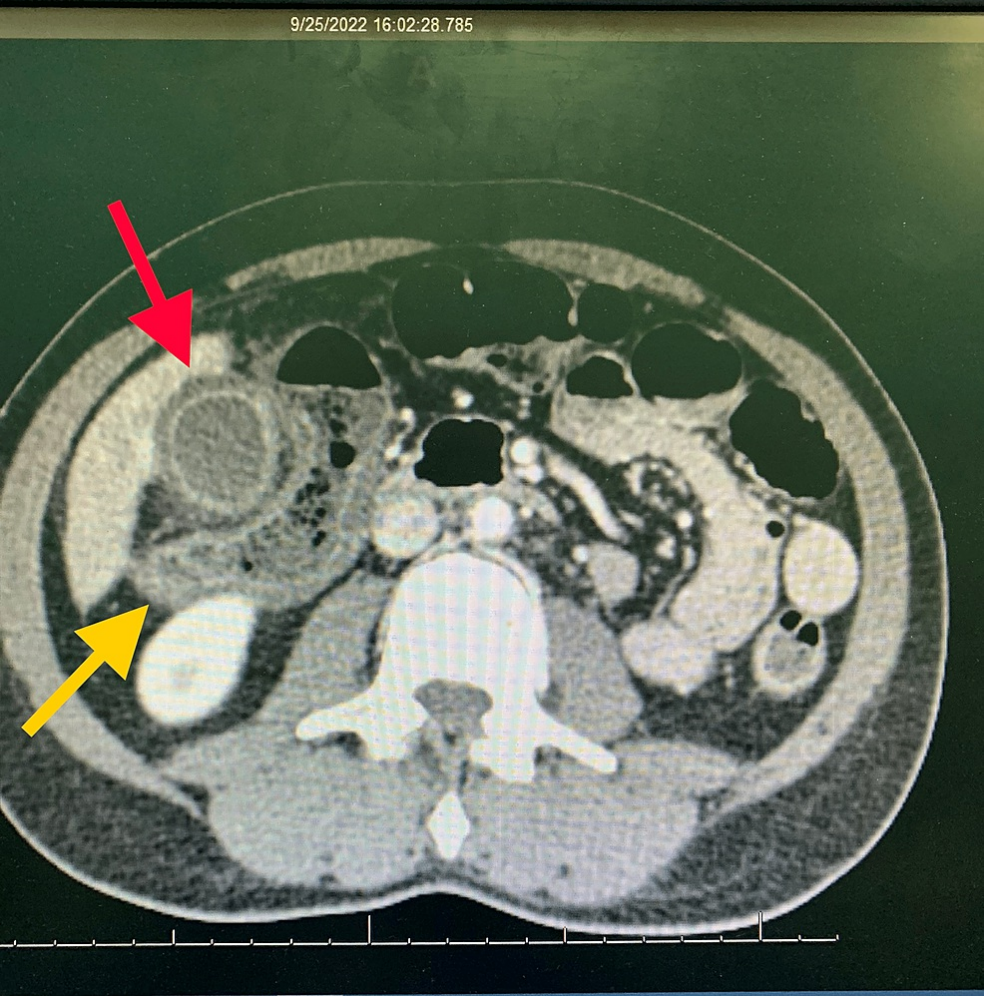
Contrast-enhanced computed tomography showing a severely inflamed gallbladder (red arrow) and inflamed hepatic flexure of the colon (yellow arrow).
